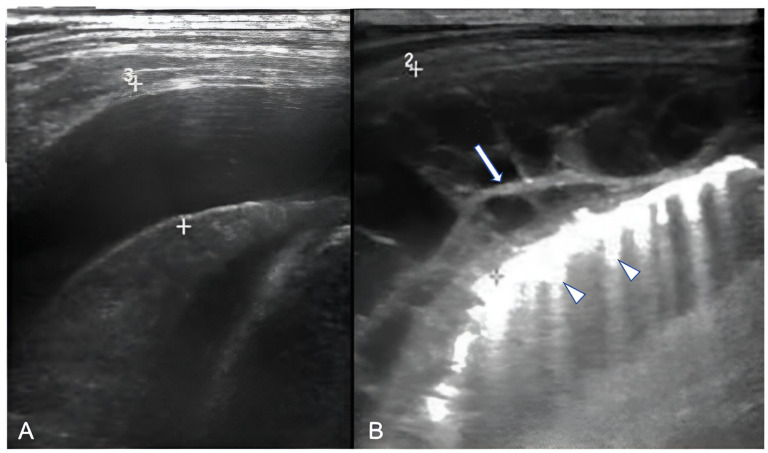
Ultrasonographic appearances of pleural effusions between cursors: (A) simple (anechoic), amenable for drainage under the appropriate clinical setting; (B) complicated, with internal septae (arrow). Also, note confluent B-lines (arrowheads).
